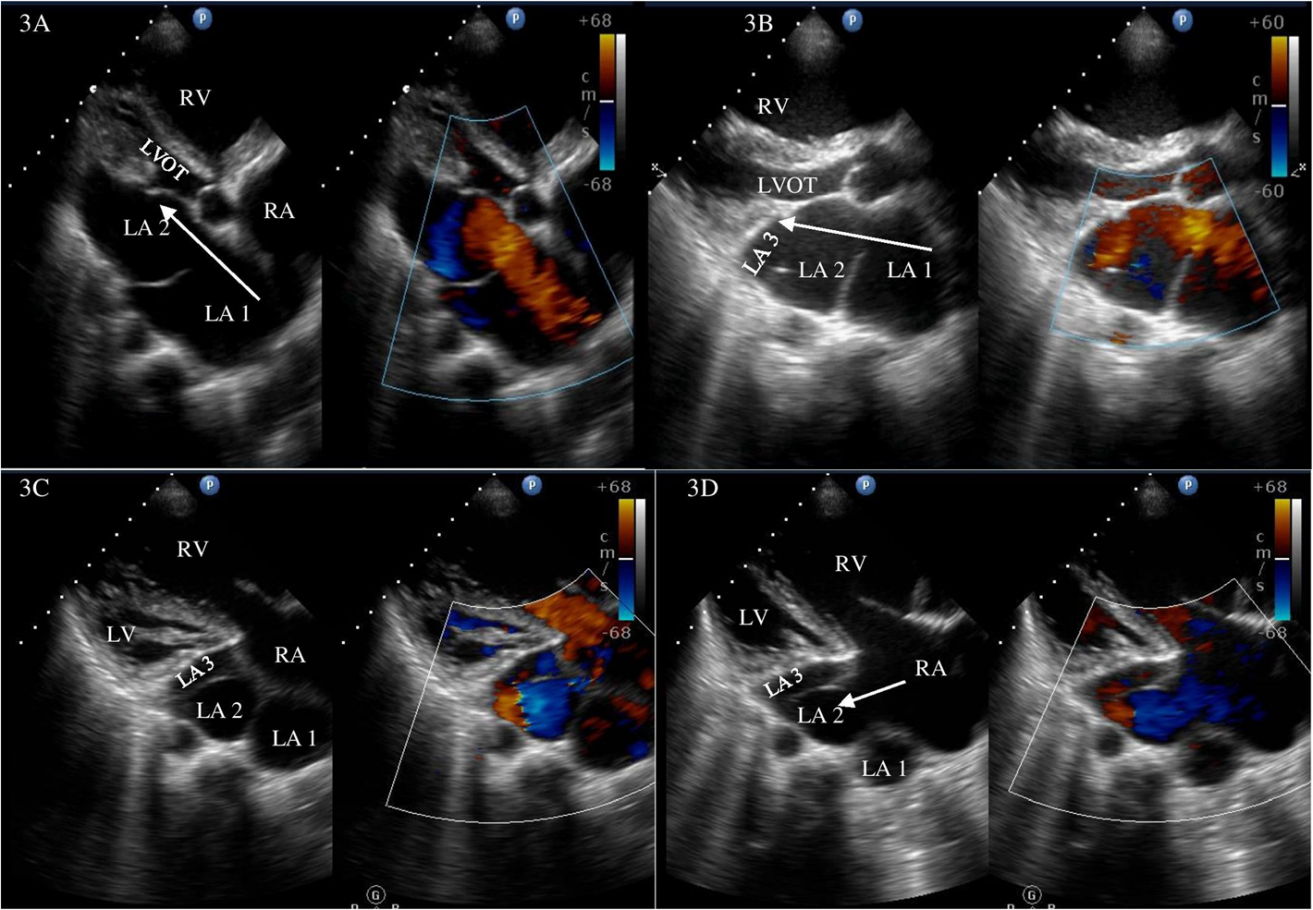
Transthoracic echocardiography in parasternal long axis (PLAX) view showing left atrium (LA) divided into 2 chambers by a non-obstructive membrane with color Doppler showing uninterrupted blood flow from LA1 to LA2 (A). Modified PLAX view showing 2 membranes in the LA diving it into 3 chambers with uninterrupted blood flow from LA1 to LA3 (B). Arrow in A and B showing the direction of flow of blood. Modified PLAX view showing all 3 chambers in LA with presence of ASD between LA2 and right atrium with predominant right to left shunt (C, D). Arrow in D shows the right to left shunt. RA, right atrium; LA, left atrium; RV, right ventricle; LV, left ventricle; LVOT, left ventricular outflow tract
